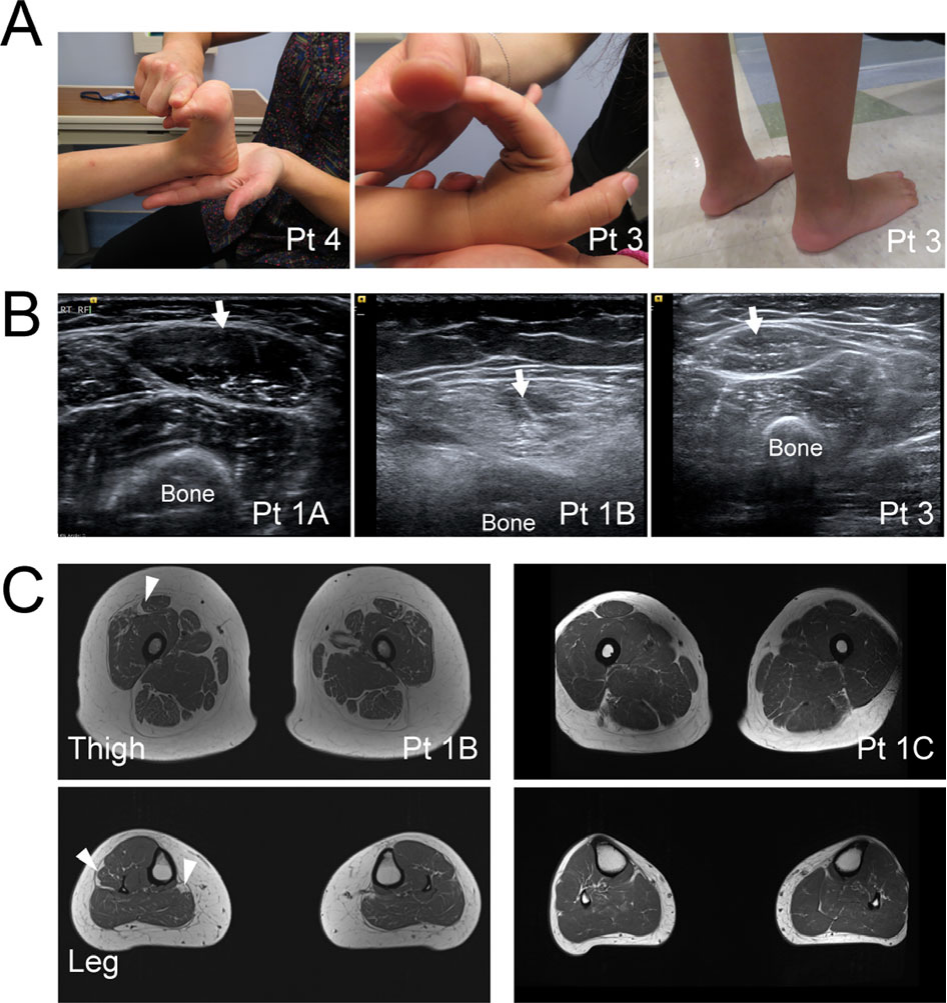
Clinical photos and muscle imaging. (A) Joint hyperlaxity (left and middle) and prominent calcanei (right) are common findings in patients with connective tissue disease, including dominant collagen XII‐related myopathic EDS. (B) Muscle Ultrasound shows normal appearing muscle in younger patients (1A, 3) and more pronounced increase in granular echogenicity in the rectus femoris muscle (arrows) in the older patient (1B). Notably, the central increase in echogenicity, characteristic of collagen VI‐related disorders is absent (1B). (C) Muscle MRI of the thigh and lower legs show mild increase in T1 weighted signal. This is most notable near the epimysium of rectus femoris, peroneus longus, and medial gastrocnemius muscles (arrowheads).
